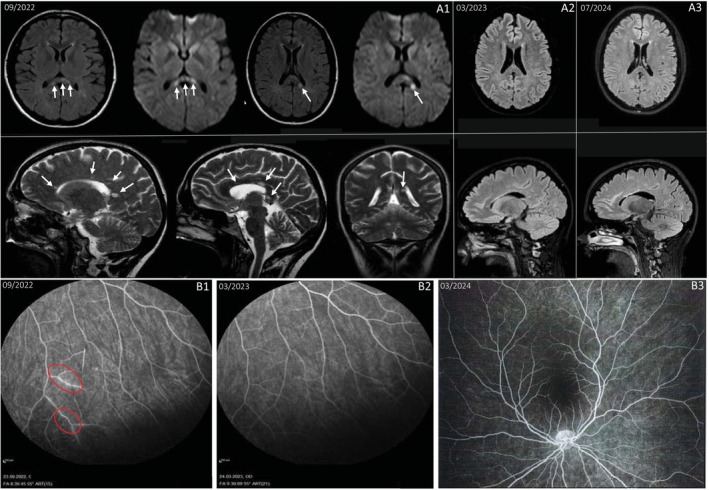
(A1) Cranial MRI scan from September 2022. Top panel: Axial Fluid‐attenuated inversion recovery (FLAIR) sequence and axial diffusion‐weighted imaging (DWI). Bottom panel: Axial and sagittal T2‐weighted turbo spin echo (T2/TSE) sequence: Multiple, predominantly diffusion‐restricted, round ‘snowball’ lesions are evident in the periventricular white matter, as well as the corpus callosum (arrows). (A2) Cranial MRI scan from March 2023 (3D FLAIR sequence). Six months following initiation of natalizumab therapy, there is an evident improvement of the previously observed lesions in the supratentorial white matter, with only smaller residual abnormalities. No evidence of blood–brain barrier disruption or new diffusion‐restricted lesions is present. No new lesions are identified. (A3) Cranial MRI scan from July 2024 (3D FLAIR sequence). Twenty two months post‐natalizumab therapy initiation, stable findings are observed with long‐term regression of supratentorial white matter lesions compared to September 2022. (B1) Fluorescein angiography from September 2022 (prior to initiation of natalizumab therapy): Vasculitic changes in the optic nerve, with areas of leakage indicated (red circles). (B2, B3) Fluorescein angiography from March 2023 and April 2024 (6 and 18 months after starting natalizumab therapy): No signs of inflammatory activity detected.
